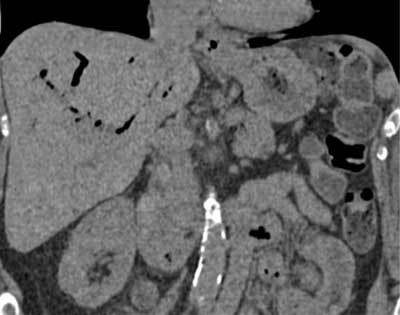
Coronal CT scan shows pneumobilia (white arrow) and common bile duct dilatation with suspicion of lithiasis (black arrow).
